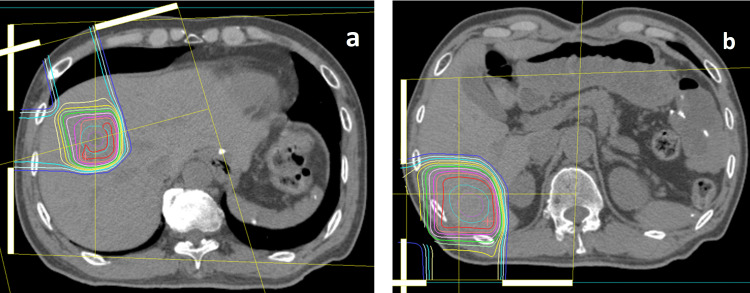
CT for the first proton beam therapy planning. The S8 (a) and S6 (b) lesions were treated by 66 GyRBE with 10 fractions for S8 tumor and 80 GyRBE with 25 fractions for S6 tumor. RBE: relative biological effectiveness
